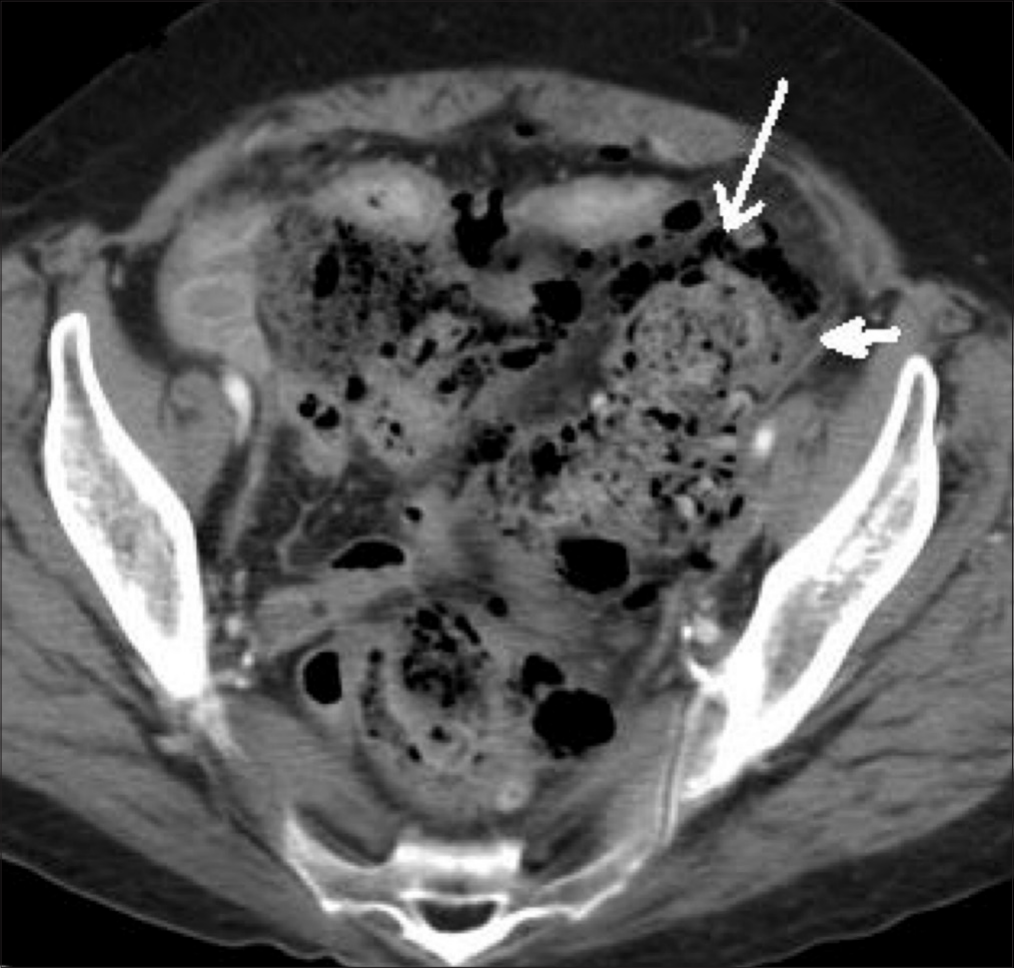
Contrast-enhanced axial CT scan demonstrates a fecaloma (arrow) in the proximal sigmoid colon and at the site of the perforation, with colonic wall thickening (arrowhead) due to pressure necrosis
